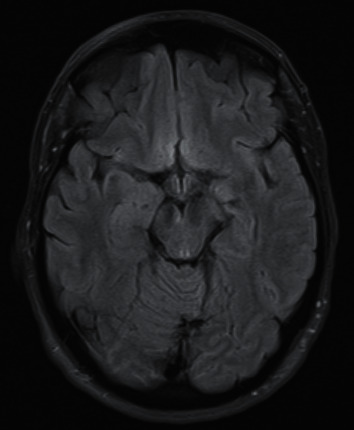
Axial FLAIR MRI showing injury in the medial aspect of the right cerebral peduncle close to the root entry zone of the right third nerve.
